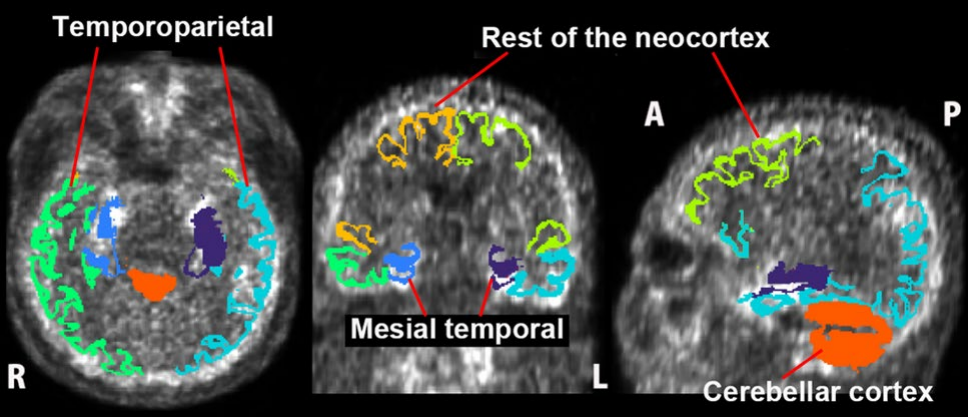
Masks for quantification of tau deposition. Three regional masks—mesial temporal, temporoparietal, and the rest of the neocortex—as well as a reference mask for the cerebellar cortex, were derived from 3 dimensional T1-weighted MRI. These masks were coregistered to tau PET scans using MRI-based native space FreeSurfer parcellations
